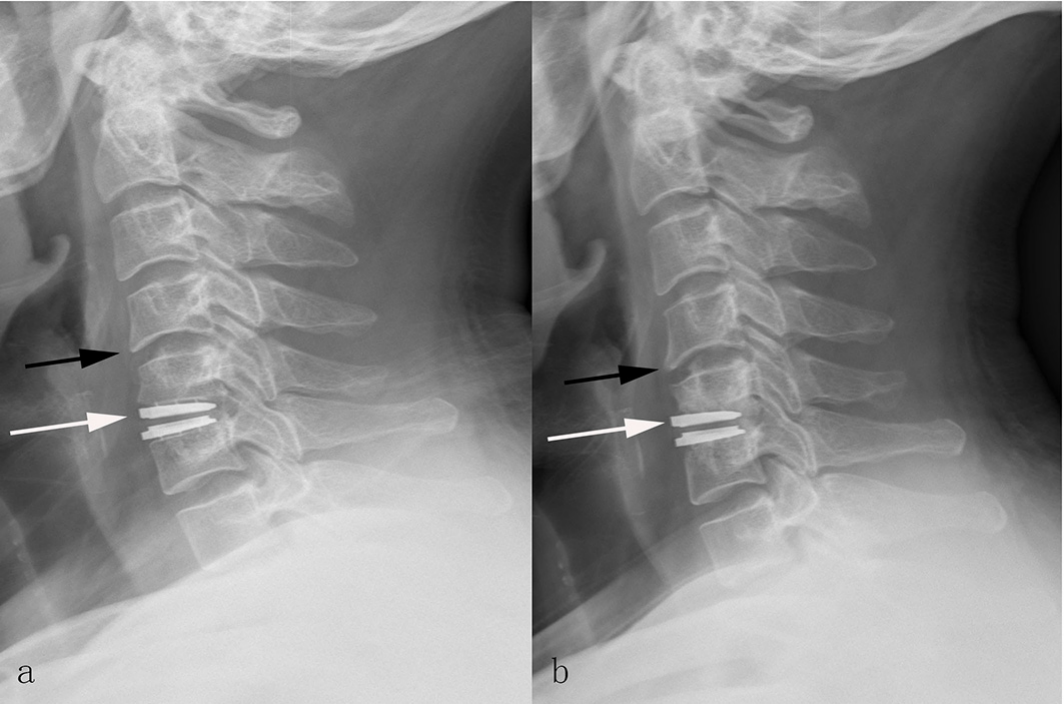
The representive case who suffered prosthesis subsidence and adjacent disc degeneration postoperatively. Postoperative 2-day (a), and final follow-up (b) lateral radiographs showing the superior endplate of Discover prosthesis subsiding into the cephalad vertebra and new anterior osteophyte formation in the patient after cervical disc arthroplasty with Discover prothesis. The white arrows indicate the subsidence of the prosthesis. The black arrows indicate the new anterior osteophyte formation.
